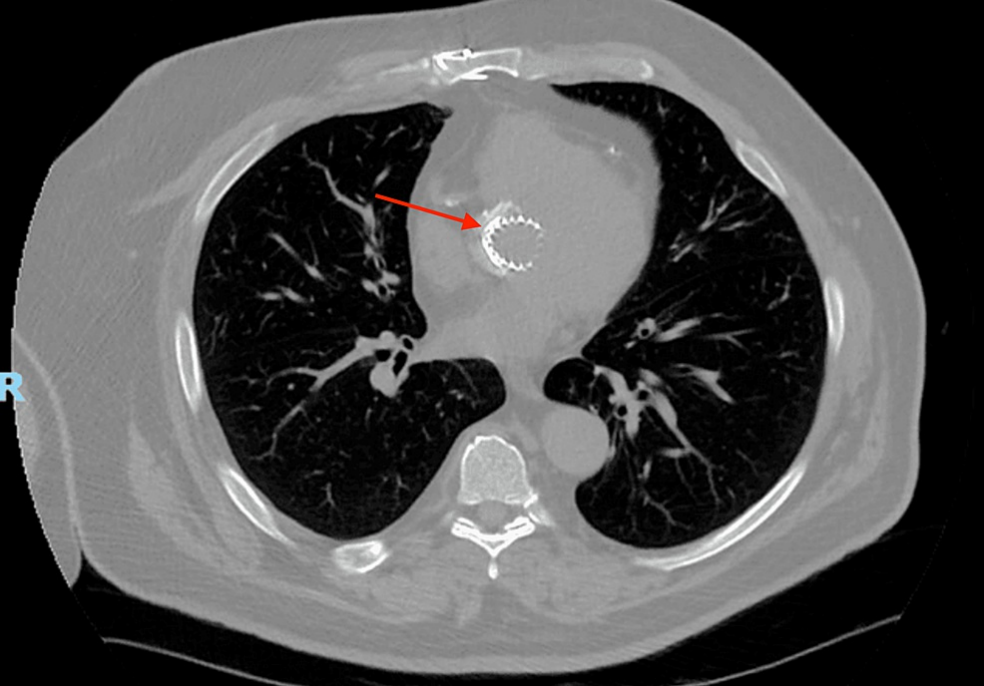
Baseline chest CT one year before COVID-19 diagnosis A computed tomography (CT) scan of the chest, conducted one year before the diagnosis of COVID-19 pneumonia, is presented herein. The axial CT image exhibits unobstructed central airways devoid of consolidations or airspace opacities. It should be noted that the patient's medical history includes a transcatheter aortic valve replacement (TAVR).
